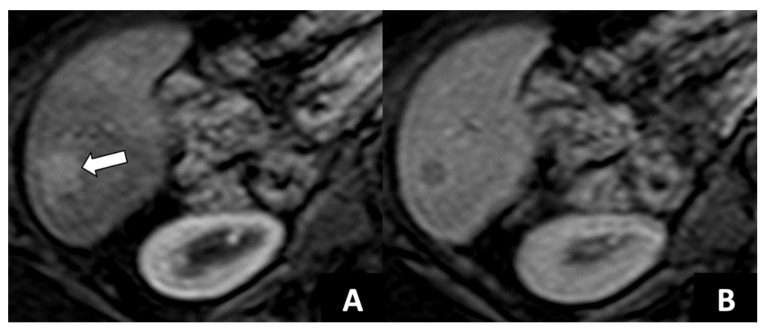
77-year-old woman with history of cirrhosis who achieved sustained virologic response with direct acting antiviral therapy. Contrast-enhanced MRI shows (A) a 1.2 cm observation (arrow) with nonrim arterial phase hyperenhancement, and (B) nonperipheral washout on portal venous phase, consistent with de novo hepatocellular carcinoma.
